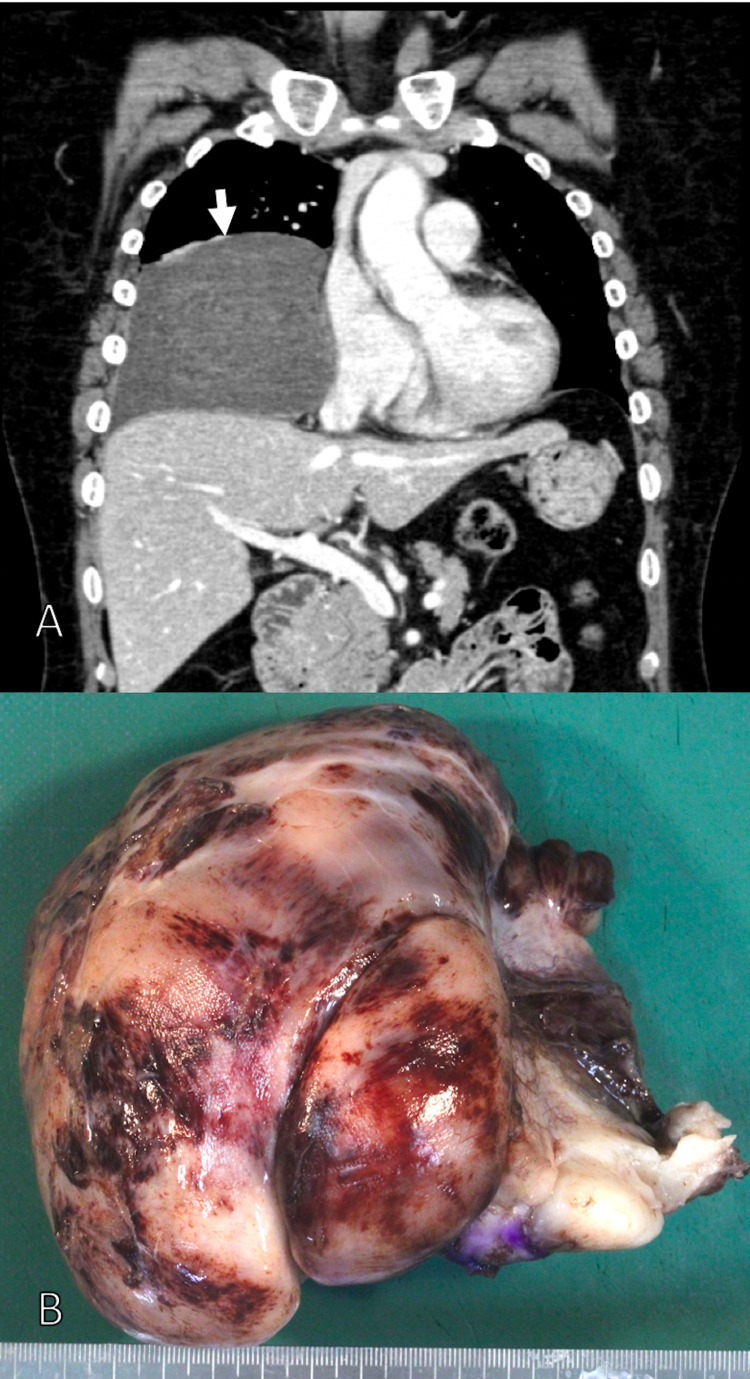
(A) Contrast-enhanced CT revealed tumor (arrows), which excluded the right diaphragm and lungs. (B) Macroscopic findings of the tumor.
